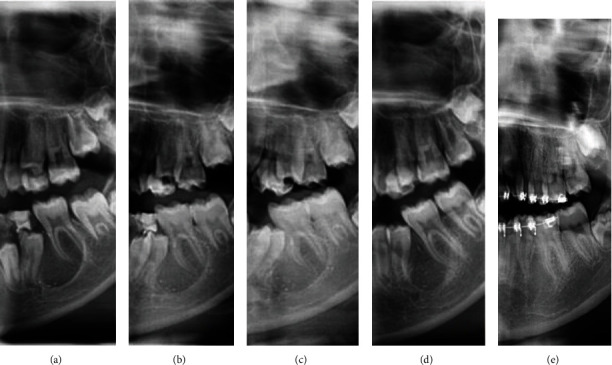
shows panoramic radiographs taken at initial and subsequent follow-up visits (a). The preoperative imaging referred by the orthodontist. (b) The lesion one-month post-intervention (c) At 3-month follow-up post-intervention. (d) At nine-month follow-up. (e) At 18 months of follow-up post-intervention showing complete resolution.
